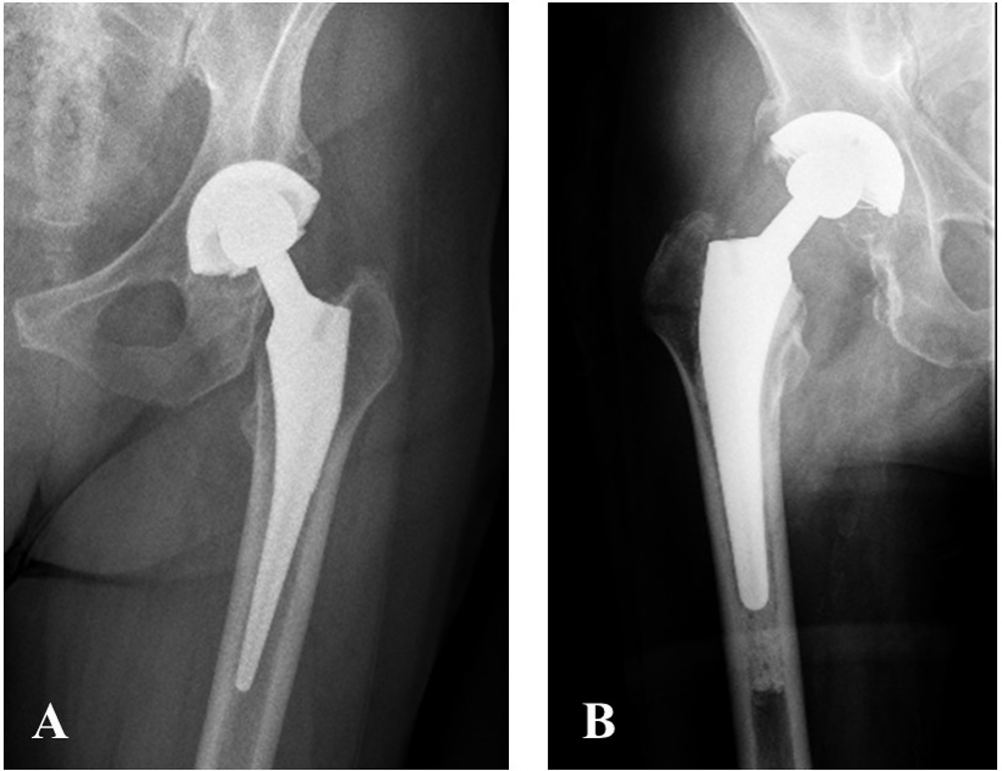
A) A 13-year follow-up radiograph of the left hip of a female patient with a cementless Taperloc stem. B) A 12-year follow-up radiograph of the right hip of a male patient with a cemented Taperloc stem.
